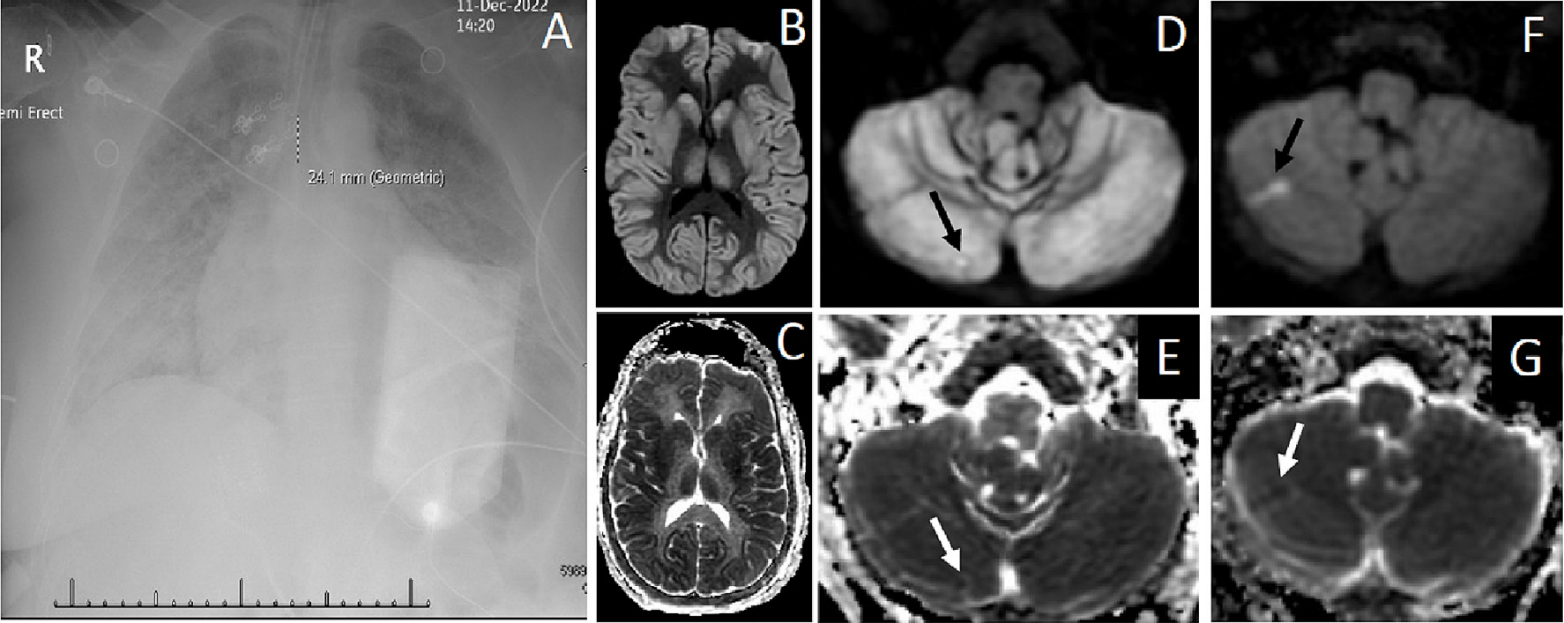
Neurogenic pulmonary edema, anoxic brain injury and recurrent ischemic infarctions in a near SUDEP patient. A: Chest X-ray taken shortly after ED arrival following a witnessed GTCS that culminated in cardiac arrest. The image shows florid bilateral postictal neurogenic pulmonary edema. The letter R in the X-ray image refers to the patient’s right side. B-G: Diffusion weighted imaging and apparent diffusion coefficient correlates demonstrating diffuse anoxic brain injury (B-C) and two small cerebellar ischemic infarctions, one diagnosed during the patient’s near-SUDEP hospitalization (D-E) and one during an earlier hospitalization, sixteen months earlier, for a presumed GTCS (F-G). Note the adjacent location of the two ischemic infarctions within the same cerebellar vascular territory. Black and white arrows show the ischemic infarctions’ DWI and ADC signals, respectively.
